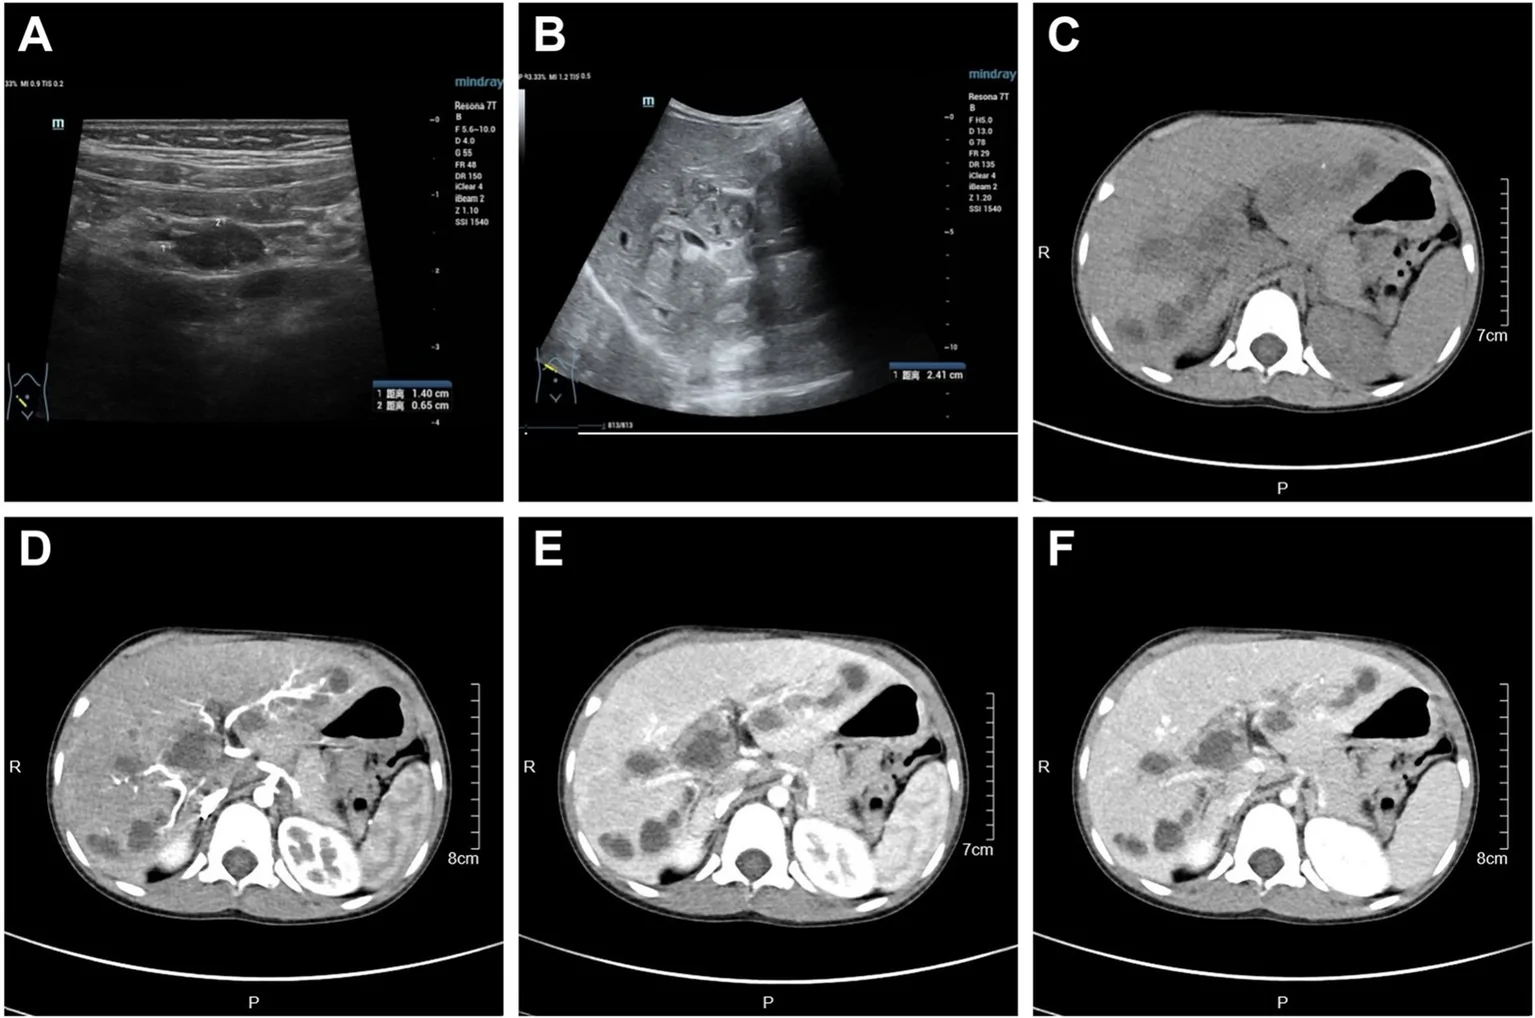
Pre-transplant imaging. (A,B) Ultrasonography on 21 November 2024 showed nodular hypoechoic hepatic lesions and multifocal cystic dilatation of intrahepatic bile ducts with wall thickening. (C–F) Contrast-enhanced CT on 22 November 2024 showed marked intrahepatic biliary dilatation and enhancing thickened bile duct walls across dynamic phases, supporting the initial radiologic impression of Caroli syndrome.
